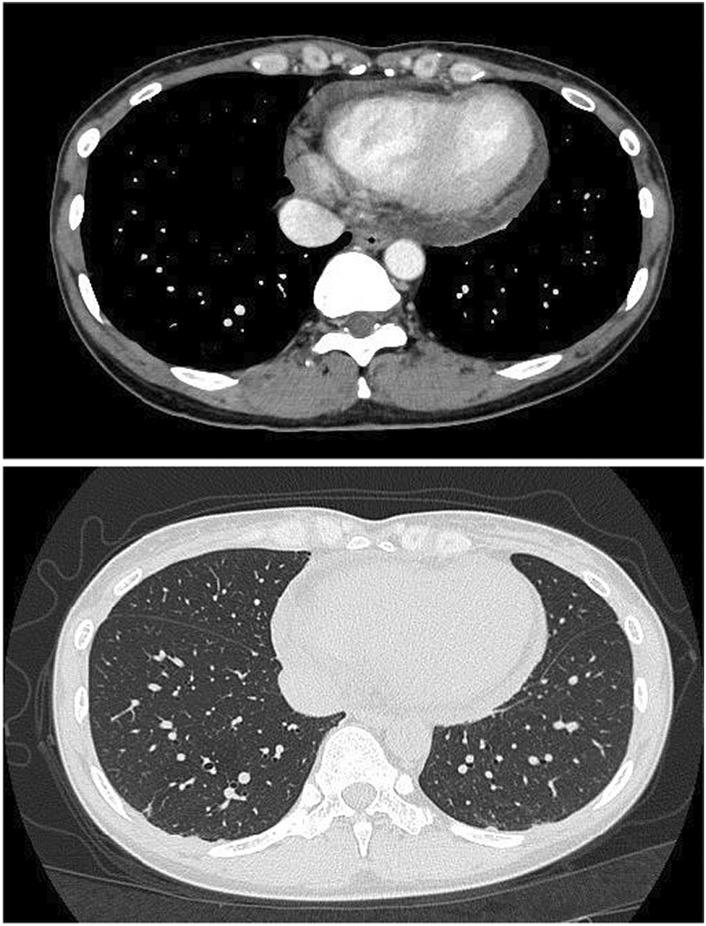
Chest computed tomography on admission showing significant pericardial effusion without infiltrates in both lungs.
